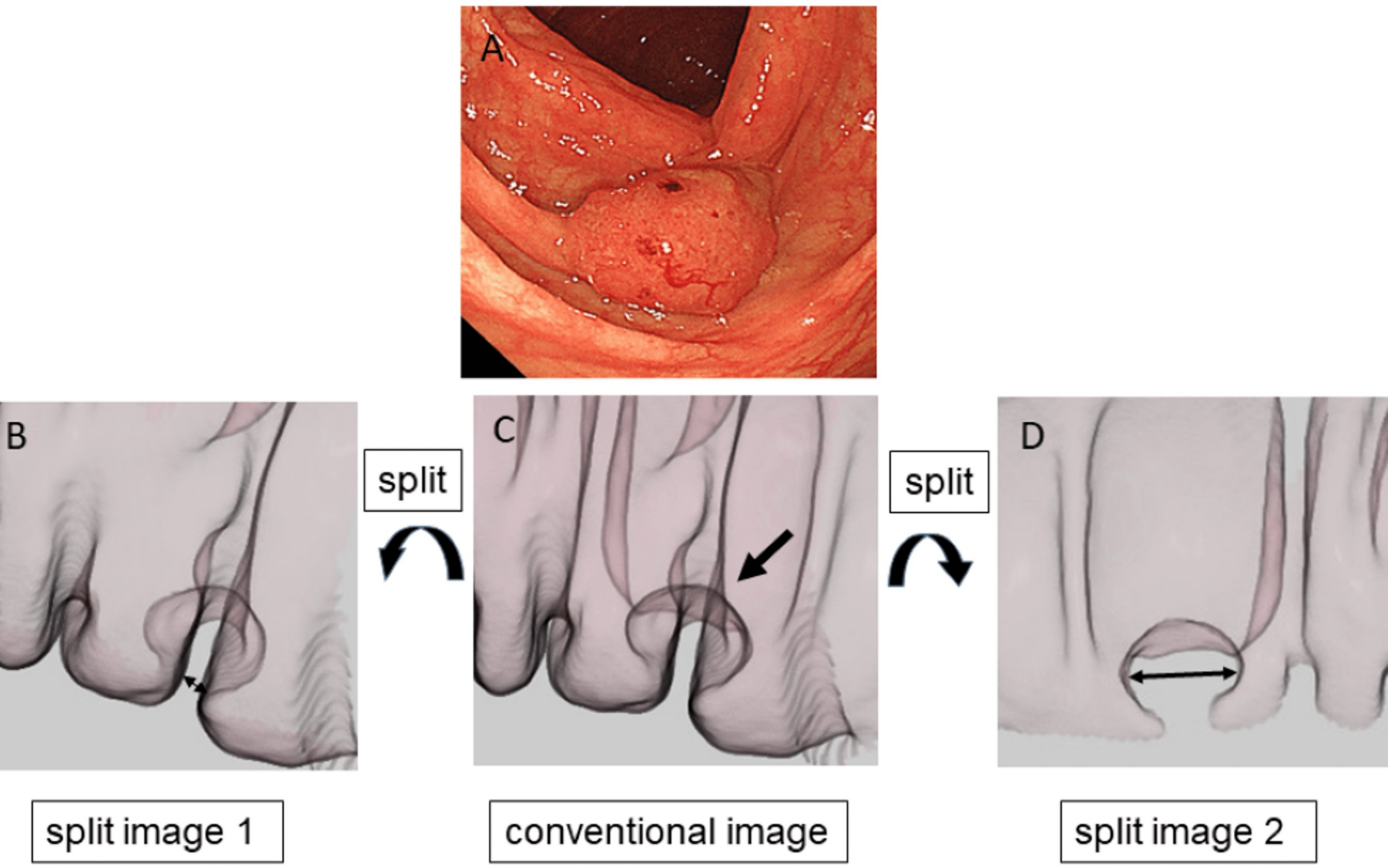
A 57-year-old woman with T1b-stage ascending colon cancer, type 0-Is. (A) Optical endoscopic image shows an elevated lesion located on a colorectal fold. (C) The lateral view of the conventional CT enema image. The values of a width (double arrow) of colorectal-fold lateral contour on the lateral split-view CT enema images 1 (B) and 2 (D) in the CRC located on a colorectal fold (arrow) were 2 mm and 11 mm, respectively. Accordingly, the maximum value of the width of colorectal-fold lateral contour was diagnosed as 11 mm. This figure was created by the authors for this study. CRC: colorectal cancer; CT enema: computed tomographic air-contrast enema
